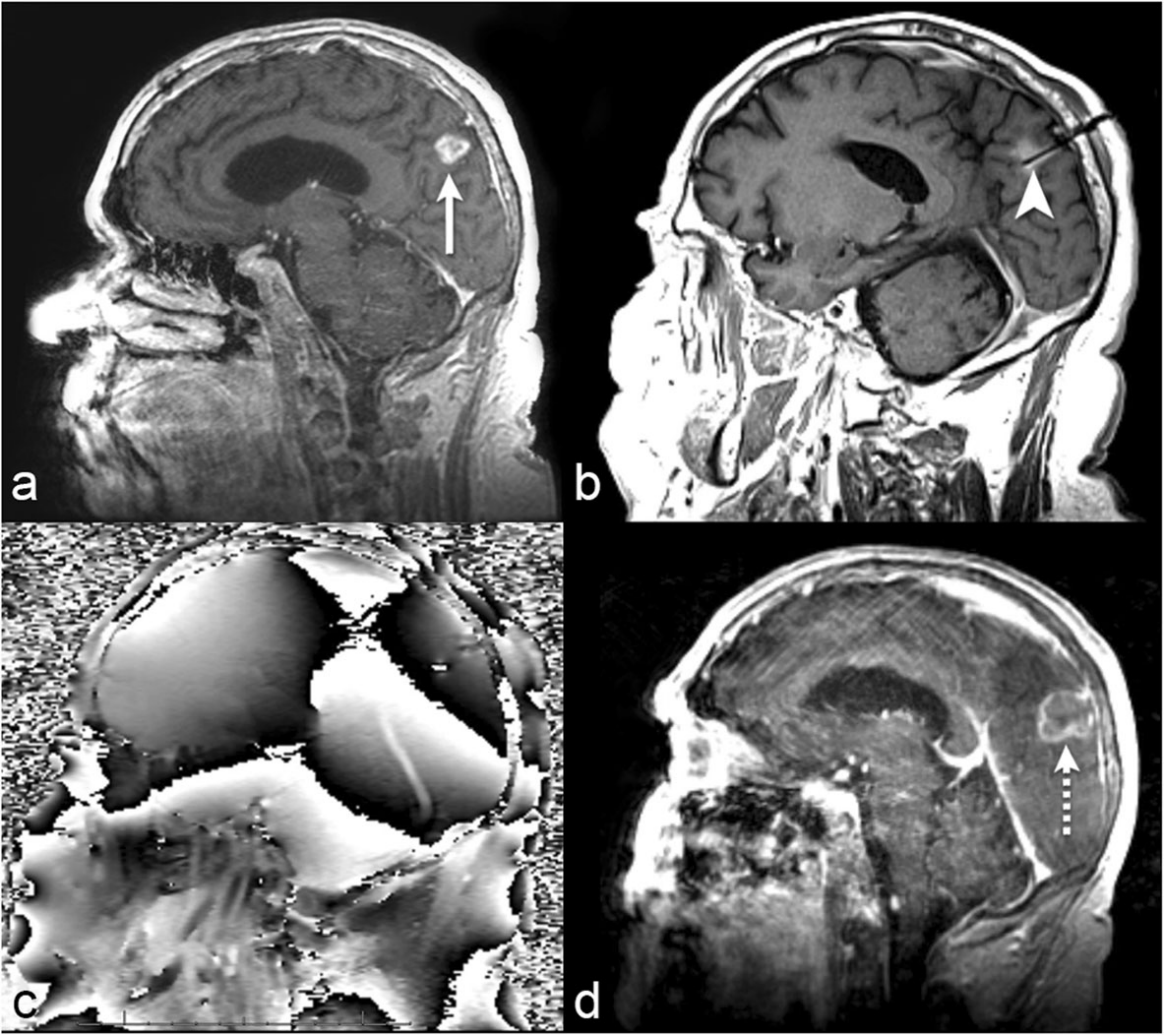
72-year-old with metastatic melanoma. a Sagittal post-contrast T1-weighted imaging before LITT demonstrates a progressing enhancing lesion in the left inferior parietal lobule at a site of a brain metastasis previously treated with gamma knife radiation therapy (long arrow). The lesion was biopsied intraoperatively immediately before LITT and was found to represent radiation necrosis. b Sagittal intraoperative localizing T1-weighted imaging with the laser probe within the lesion (arrowhead). c Sagittal intraoperative gradient-echo phase imaging is the source of the thermography maps. By subtracting subsequent images during heating from a reference image acquired before heating, a map of temperature change can be formed. d Sagittal post-contrast T1-weighted imaging 1 month after LITT demonstrates complete ablation of the lesion (dashed arrow)
